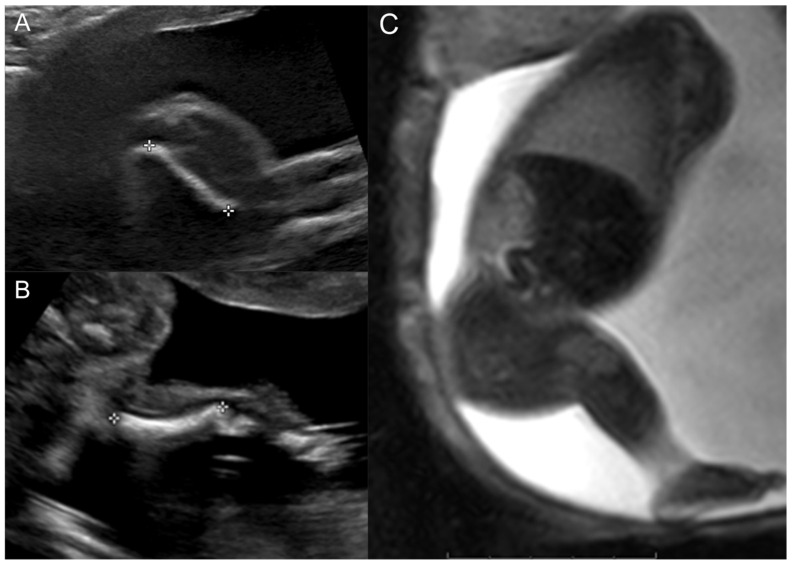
Prenatal right lower extremity examination at 23 weeks gestation. (A) Right lower extremity US demonstrating short femur. (B) Right lower extremity US demonstrating the presence of a single bone in the leg (tibia) and absent fibula. (C) Thick slab balanced turbo field echo MRI images of the entire right lower extremity.
